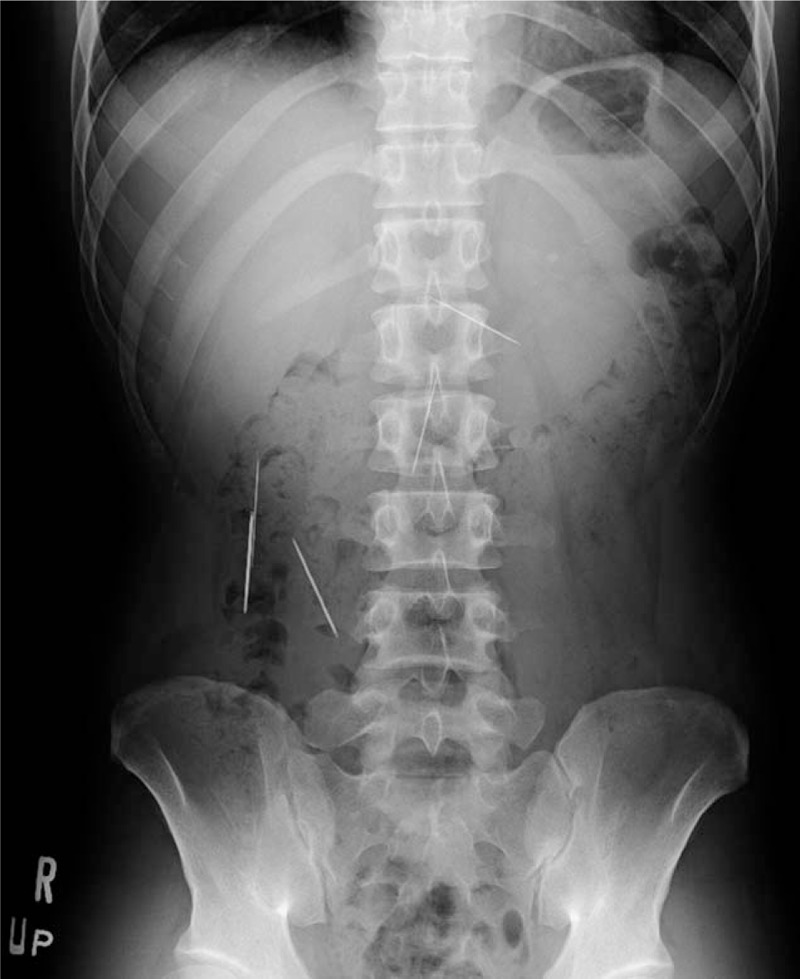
Abdominal radiograph showed multiple sewing needles in the abdominal cavity.
